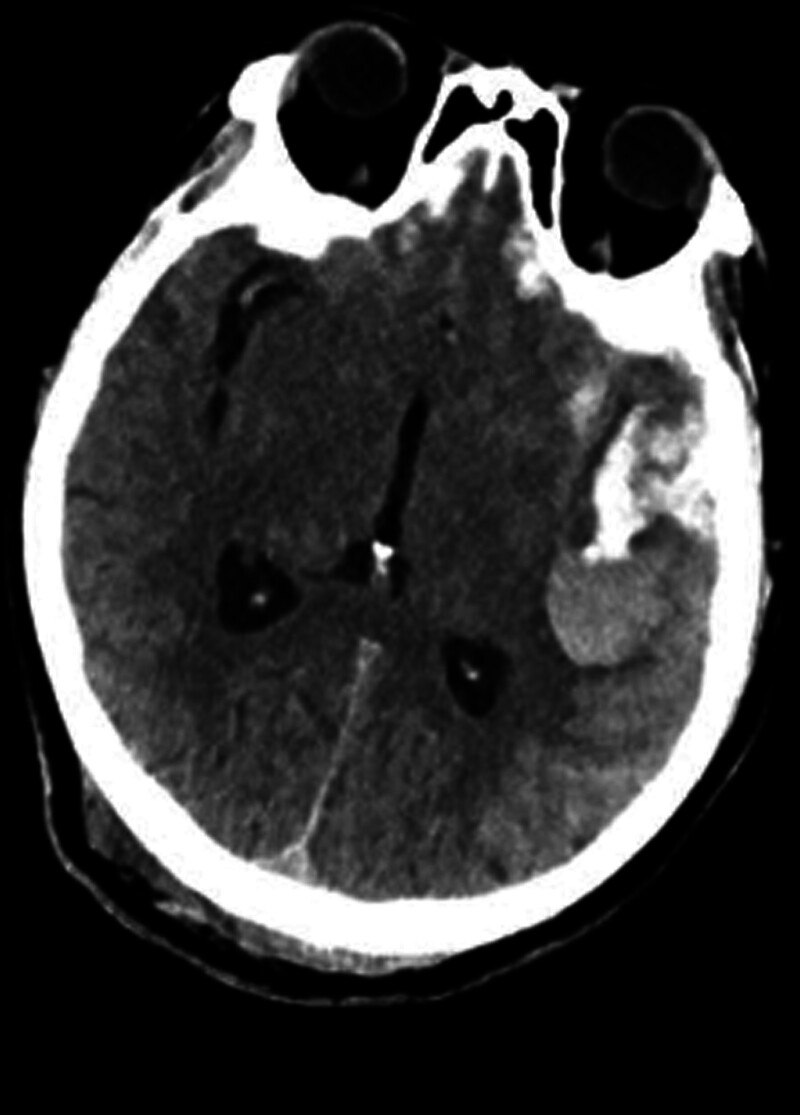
Head CT: compared with the morning head CT scan, the current hematoma has slightly increased in size and shows higher density. The subarachnoid hemorrhage remains unchanged. A pre-herniation state is suspected. CT = computed tomography.
